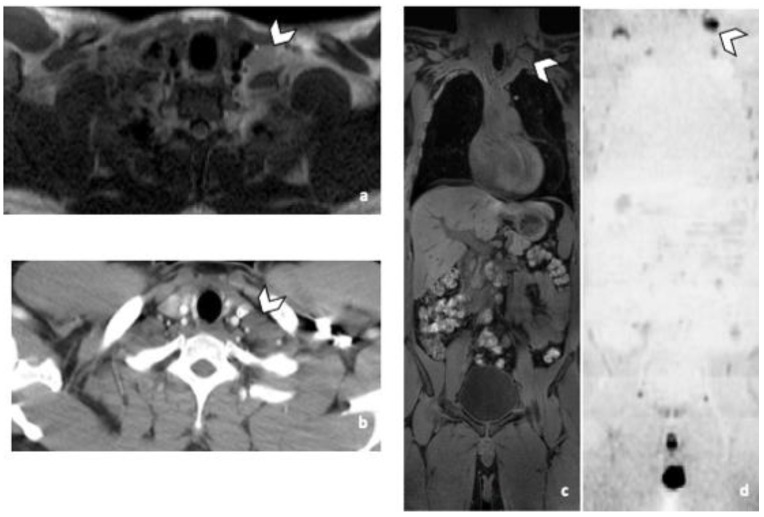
False negative supra-diaphragmatic lymph node during the CT reading in a 28-year-old man with NSGCC. Axial T2-weighted image (a), coronal reformatted 3D T1 GRE Fat Sat (c) and high b-value DWI (inverted greyscale, b = 1000 s/mm^2^) MR images of the whole body (d) and axial CT image after intravenous injection of iodinated contrast agent (b). The enlarged left supra-clavicular lymph node (arrowhead) was identified by both readers during the MRI readings; it was missed by both readers during the CT readings. Abbreviations: NSGCC: Non-Seminomatous Germ Cell Cancer, 3D: Three Dimensional, T1 GRE: T1 Gradient Echo, DWI: Diffusion Weighted Imaging, MRI: Magnetic Resonance Imaging, CT: Computed Tomography.
